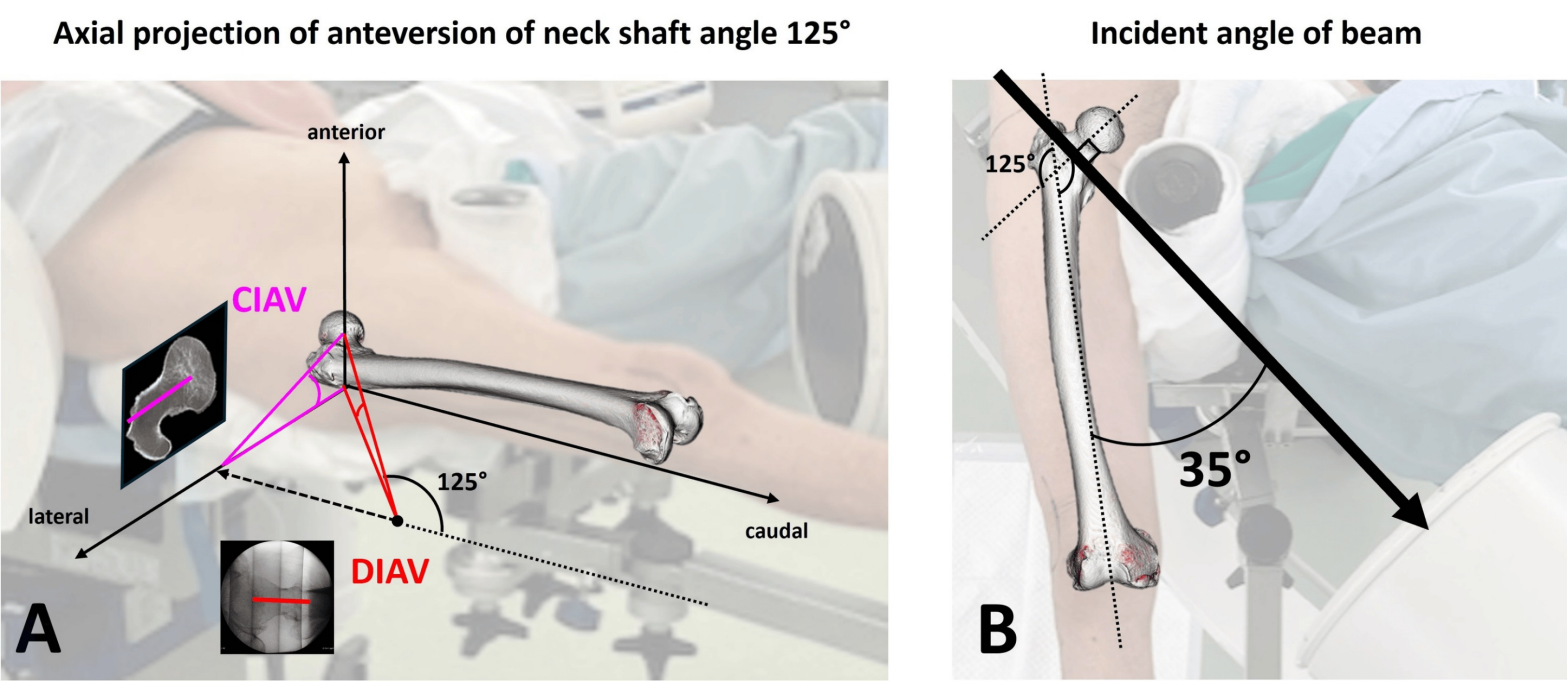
Axial projection of anteversion on horizontal lateral hip view Direct intraoperative anteversion (DIAV) was converted into corrected intraoperative anteversion (CIAV). The CIAV refers to the value measured in the same plane as the axial section of the CT image (A). The condition of a neck shaft angle of 125° indicates that the beam of the image intensifier is set at an incident angle of 35° to the femoral diaphyseal axis and vertical to the neck axis, based on a femur with a neck shaft angle of 125° (B).
